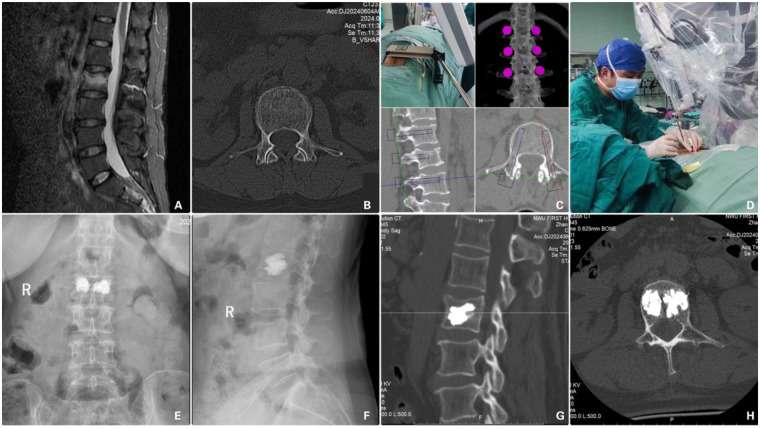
(A) preoperative MRI Fat-suppressed sequence, (B) preoperative axial CT scan, (C) intraoperative robot-assisted planning, (D) intraoperative robot-guided operation, (E,F) x-ray images on postoperative Day 2, (G,H) CT images on postoperative Day 2.
